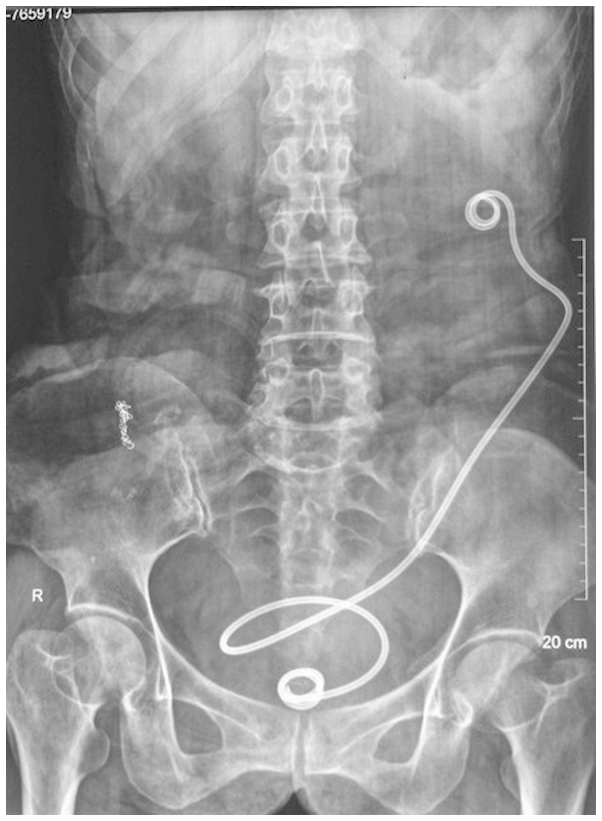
A representative kidney, ureter and bladder X-ray film following the subcutaneous nephrovesical bypass (SNVB) procedure. The SNVB bypass was implanted for a left ureteral obstruction and an appropriate site was chosen. The upper curved part of the bypass is located in left kidney, with the lower part in the bladder.
